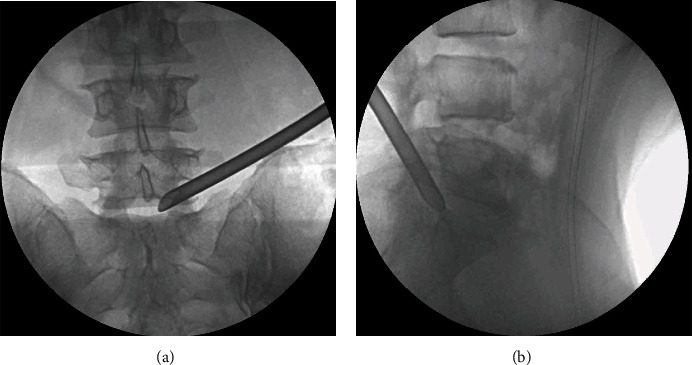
Final position of the working sheath confirmed with fluoroscopy: (a) anteroposterior radiograph; (b) lateral radiography.
